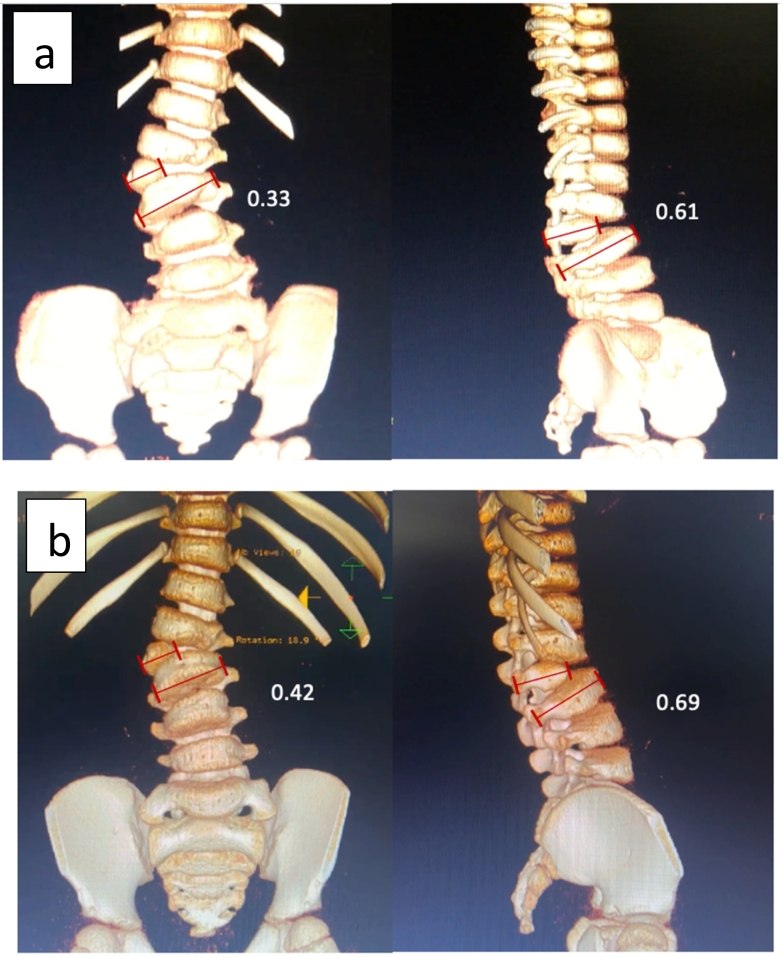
Comparison of 3D CT between a) before MSCs implantation and b) 3 years after MSCs implantation. The hemivertebra ratio was conducted from the maximum horizontal hemivertebra length and the maximum horizontal normal vertebra length (showed in red line). On the anterior view, the ratio increased from 0.33 to 0.42 whereas on the lateral view, the ratio increased from 0.61 to 0.69. (For interpretation of the references to colour in this figure legend, the reader is referred to the web version of this article.)
